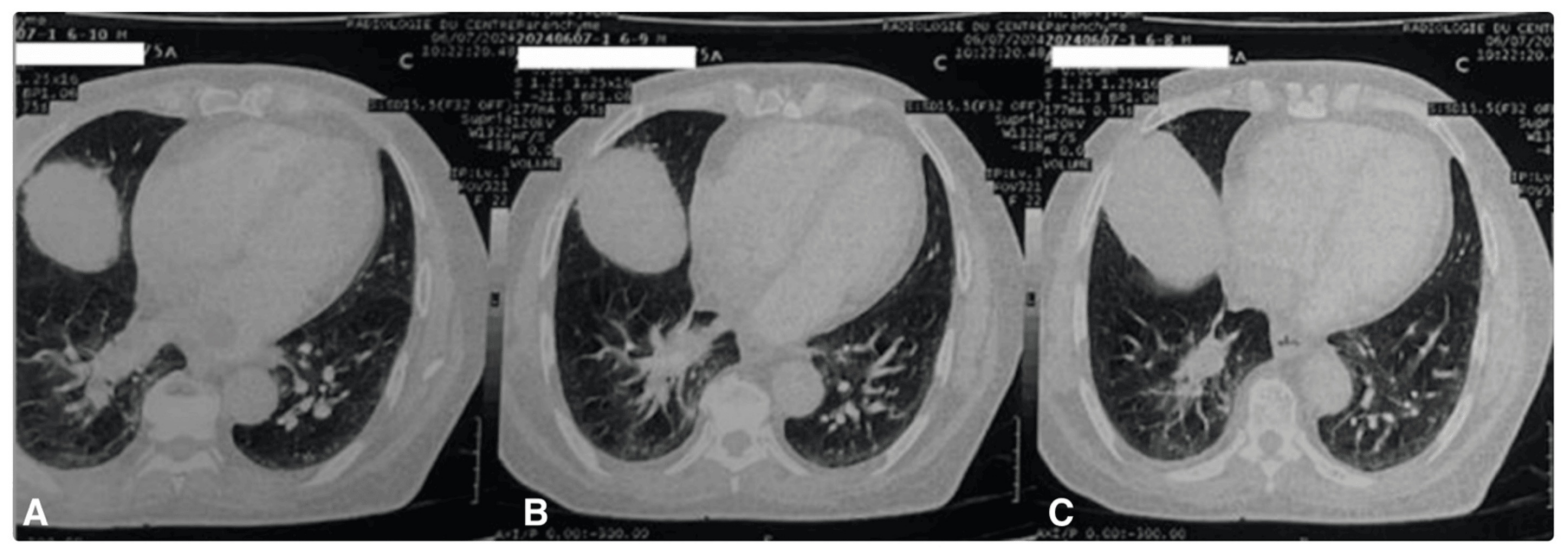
Chest CT in parenchymal window demonstrating parenchymal extension of the cardiac mass into the right lower lobe. (A) Exoluminal component of the cardiac mass extending through the right pulmonary hilum. (B) Extension of the cardiac mass into the right lower lobe parenchyma. (C) CT image revealing parenchymal involvement.
